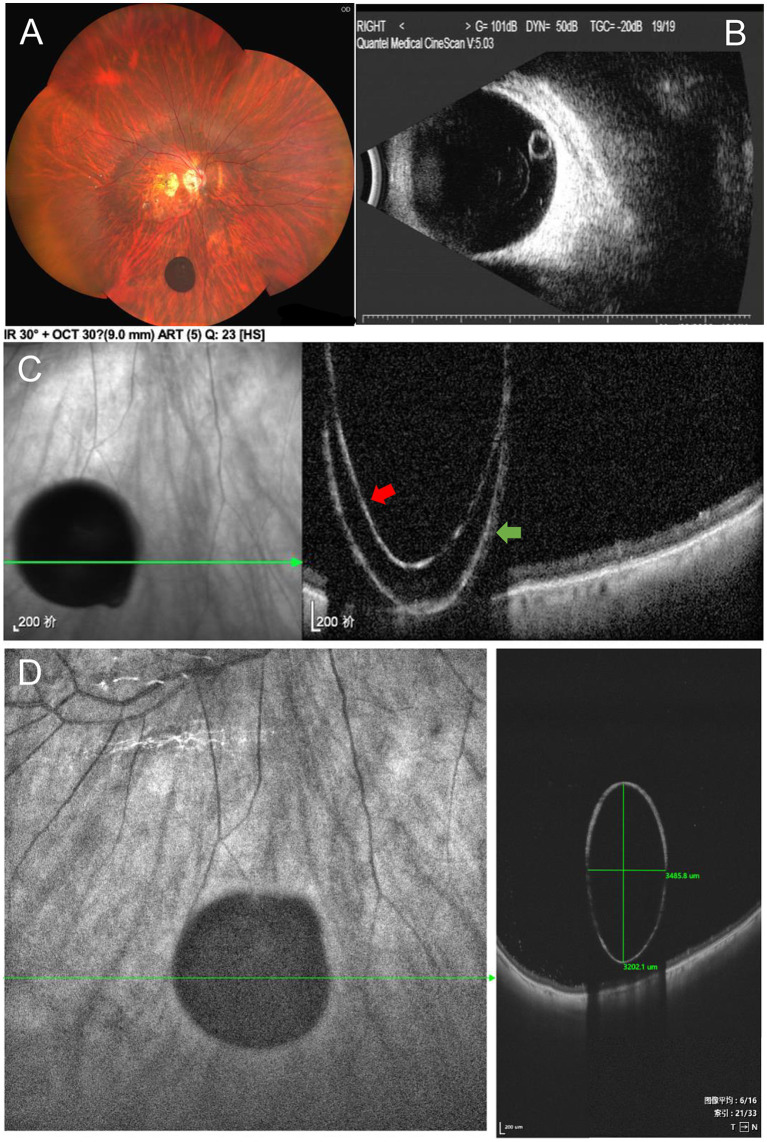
(A) Broad line fundus imaging showed a round, pigmented cyst. (B) Ultrasound B-scan showed the posterior-located vitreous cyst. (C) Spectral-domain OCT (SD-OCT) showed an image of a cyst with an inverse line. Red arrow: High-reflectivity band corresponds to the posterior edge of the vitreous cyst. Green arrow: The reflection image formed by exceeding the SD-OCT upper limit. (D) Ultra-wide field swept-source optical coherence tomography (SS-OCT), with 9 mm scan depth and 12 mm scan length, demonstrated a complete cyst image (3.5 mm × 3.2 mm in size) with a hyper-reflective enclosure, hypo-reflective content, and shadowing of the underlying retina.
